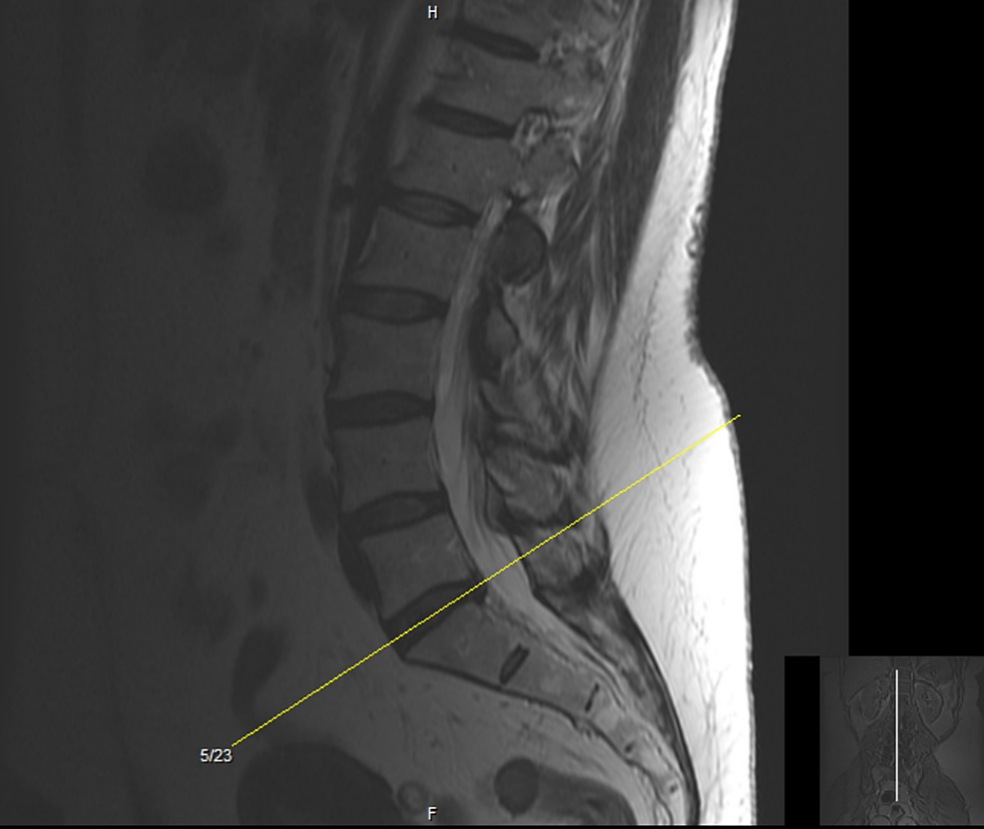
Lumbar MRI without contrast: sagittal view. MRI: magnetic resonance imaging
